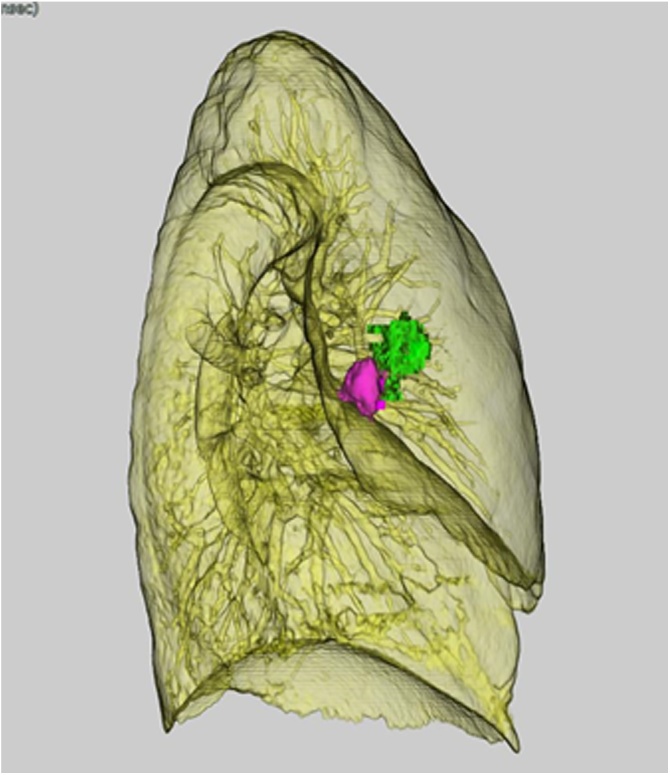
Three-dimensional construction image after a computed tomography-guided marking. Red shows a tumor, and green shows an injected site.
